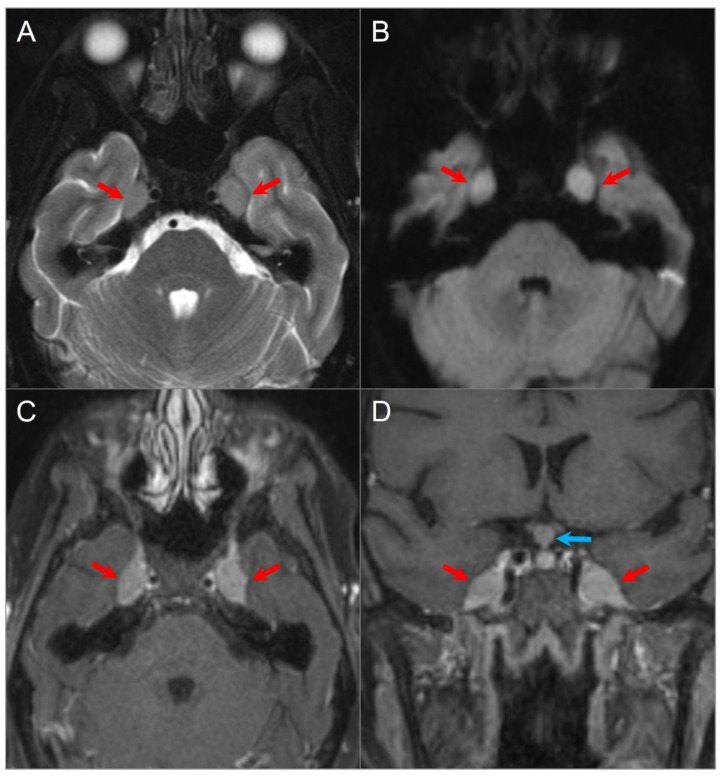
Meckel’s cave and pituitary stalk involvement of acute lymphoblastic leukemia. (A) Axial STIR T2W image shows well-defined nodular lesions in the bilateral Meckel’s caves (red arrows) with isointense signal relative to the cerebral cortex. (B) DWI image demonstrates mild restricted diffusion in the lesions. (C) Axial and (D) coronal fat-suppressed postcontrast images reveal homogeneous enhancement of the lesions. There is a nodular lesion in the pituitary stalk with similar signal characteristics (blue arrow).
